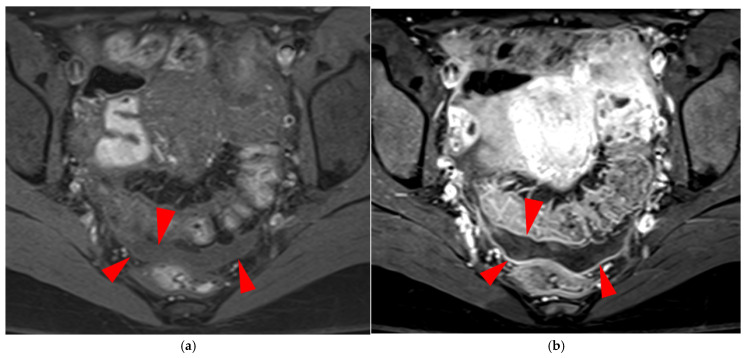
Pelvic MRI scan of a patient with likely superficial endometriosis, performed during a painful menstrual period. (a) Axial fat-suppressed T1WI demonstrates moderate dependent peritoneal effusion (*arrowheads*). (b) Axial contrast-enhanced fat-suppressed T1WI reveals perceptible curvilinear enhancement of the peritoneal layers, suggesting the possible presence of superficial endometriotic lesions (*arrowheads*).
